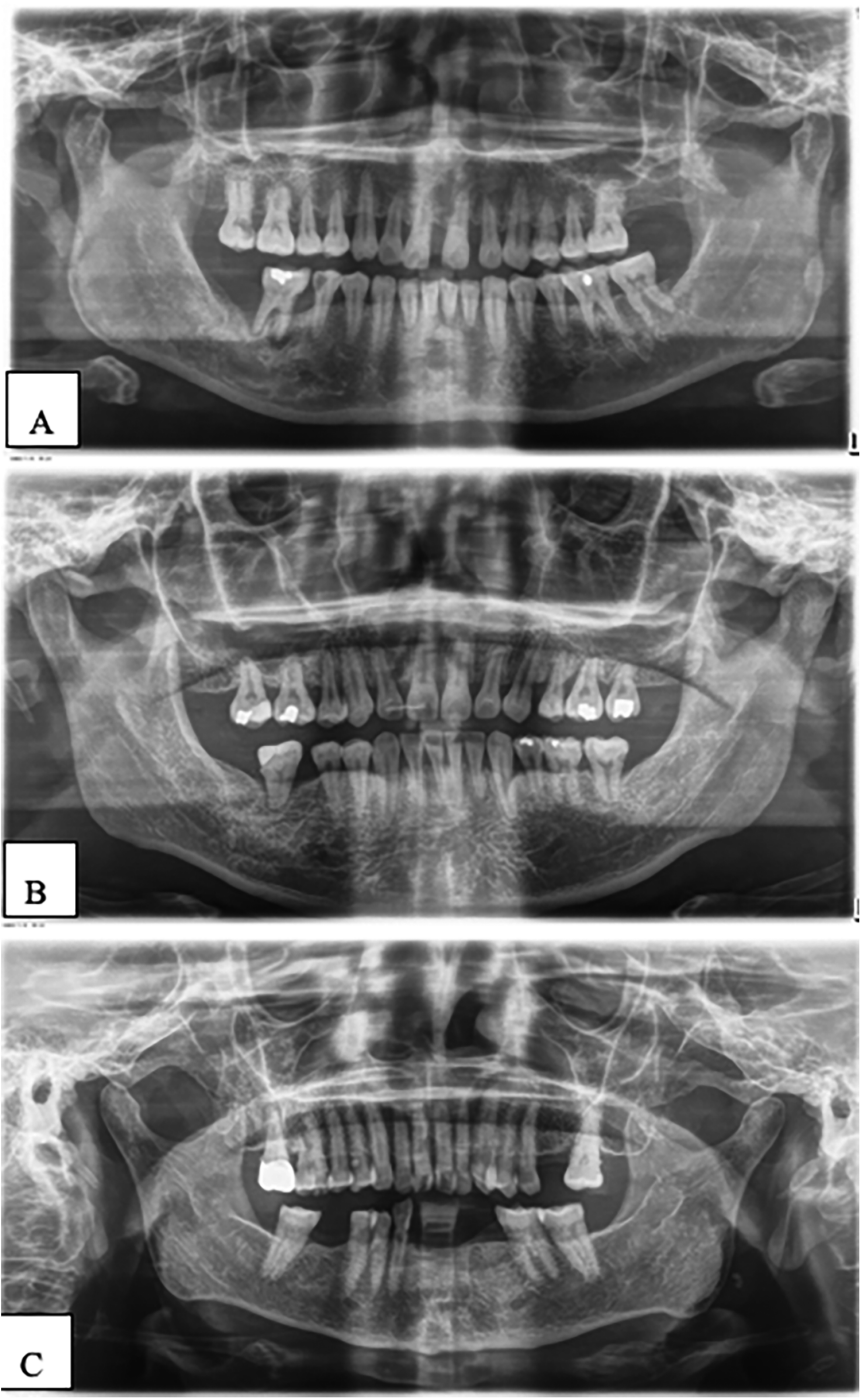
(A–C) Examples of panoramic radiographs captured using the ORTHOPHOS XG device (Sirona, Bensheim, Germany).
